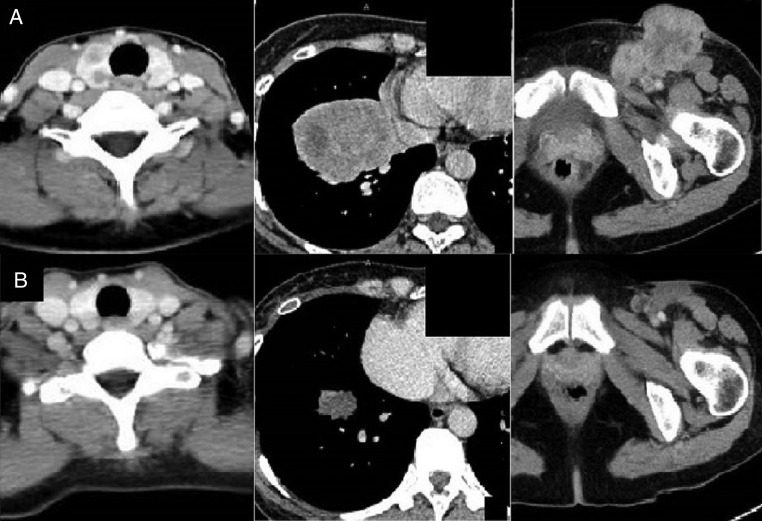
(A) CT shows the size in the right lobe of the thyroid gland, the right lower lung lobe and the left inguinal lymph node before treatment. (B) Three months after the beginning of crizotinib therapy. The tumour sizes are all reduced.
